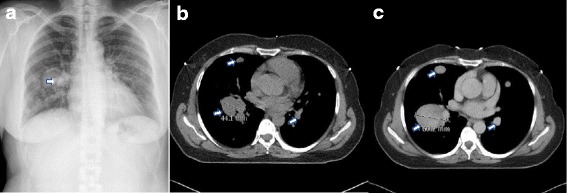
X-ray (a) and high resolution computed tomography (b, c) images of the BML patient from different examinations (November 2013, January 2015 and June 2015, respectively). Pulmonary metastases are shown by arrows
